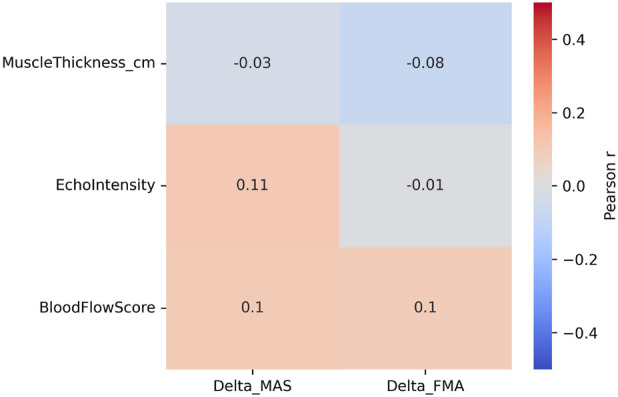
Heatmap of correlations between ultrasound and outcome measures.
